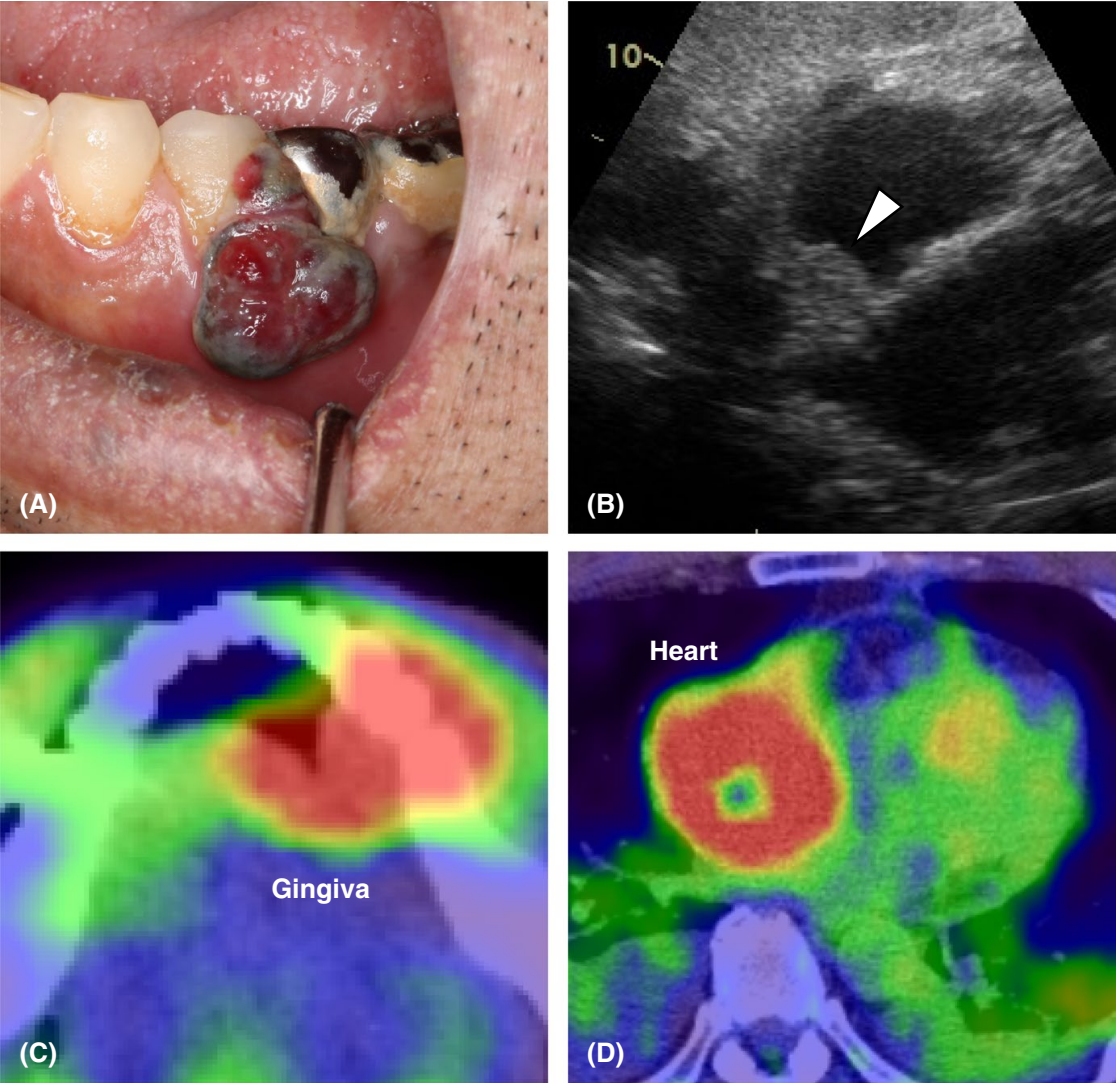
Appearance of the left lower gingival mass (A). Echocardiogram showing a mass in the right atrium (B). FDG‐PET/CT images showing a gingival to mandibular lesion on the left side (C) and a right atrial mass (D) with FDG uptake. FDG, ^18^F‐fludeoxyglucose; PET/CT, Positron emission tomography‐computed tomography.
